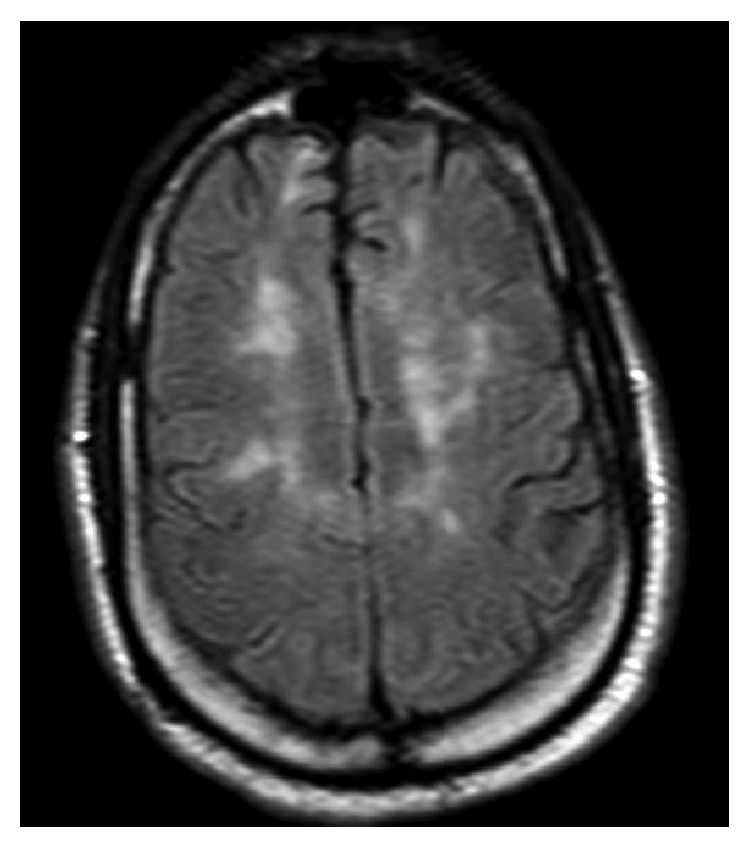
Additional subcortical hyperintensities on MRI brain T2 FLAIR sequencing.
